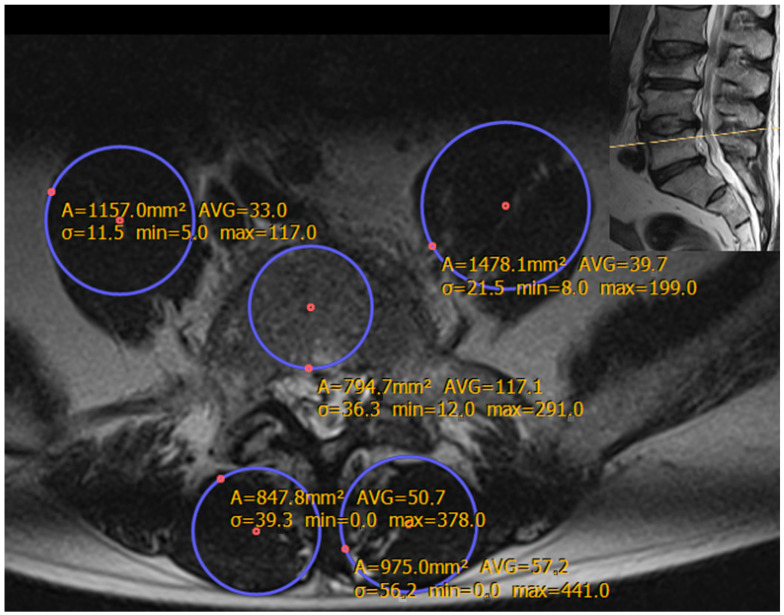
Example showing LIC area size and brightness parameters measured in the vertebral body L5, in both psoas muscles and in both posterior paravertebral muscles. Axial MRI slice in the L5-level of a 59-year-old man with a history of multiple pathologic thoracic and lumbar vertebral fractures. LIC indicates largest inscribed circle; L5, lumbar vertebra V; MRI, magnetic resonance imaging; A, area; AVG, average gray level (signal intensity, or brightness); σ, standard deviation; min, minimum signal intensity, or minimum brightness value; max, maximum signal intensity, or maximum brightness value.
